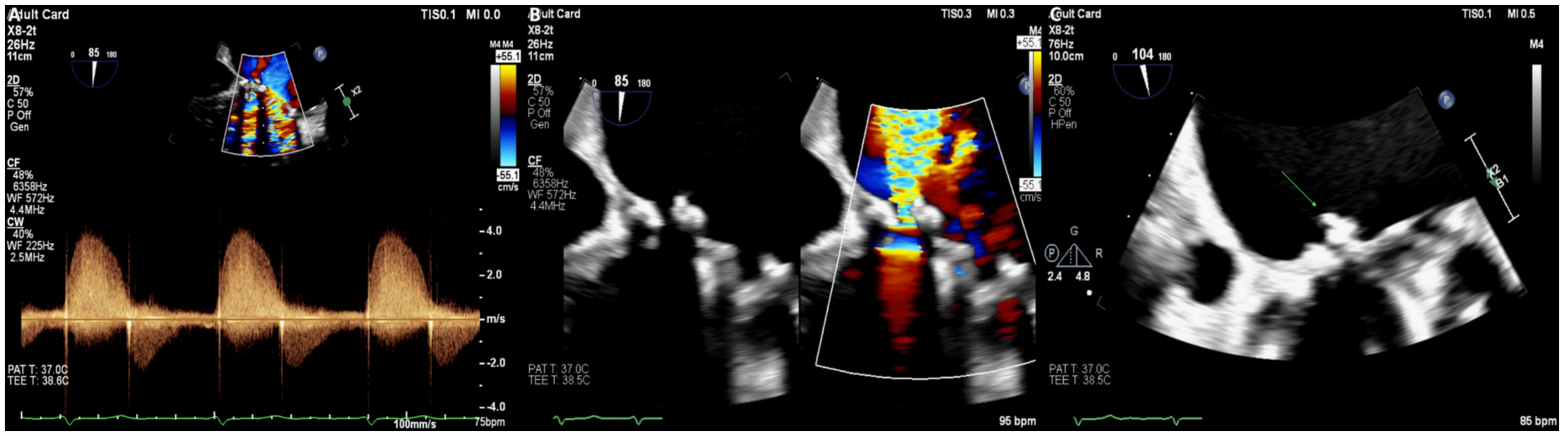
Multimodality echocardiographic assessment demonstrating severe prosthetic mitral valve involvement. (A) Continuous-wave Doppler showing dense, holosystolic paravalvular regurgitant flow. (B) Color Doppler transesophageal echocardiography revealing severe inferomedial paravalvular leak adjacent to the mechanical mitral prosthesis. (C) Transesophageal echocardiography demonstrating a small echogenic mass (arrow) consistent with vegetation at the prosthetic valve annular interface.
